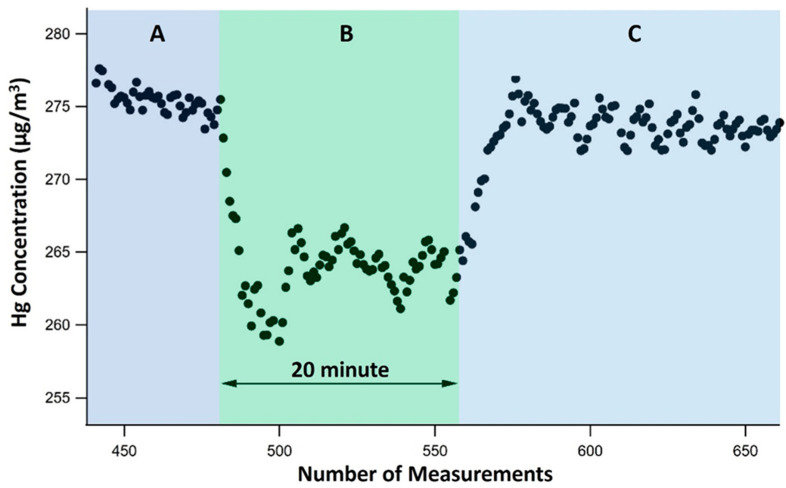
Concentration of mercury in the filtering systems. Section A: stabilization period. Section B: X-ray and power supply ON elemental Hg filtration. Section C: X-ray and power supply OFF.
